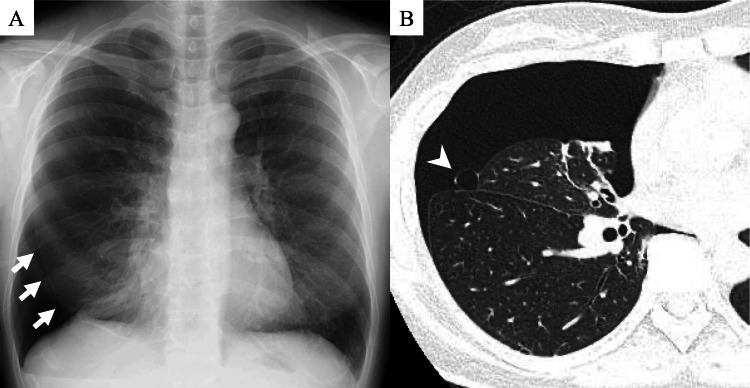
Chest X-ray (A) and chest computed tomography (B). A: Chest X-ray showing a right pneumothorax (arrow). B: Chest computed tomography showing bulla in the right middle lung lobe (arrowhead).
